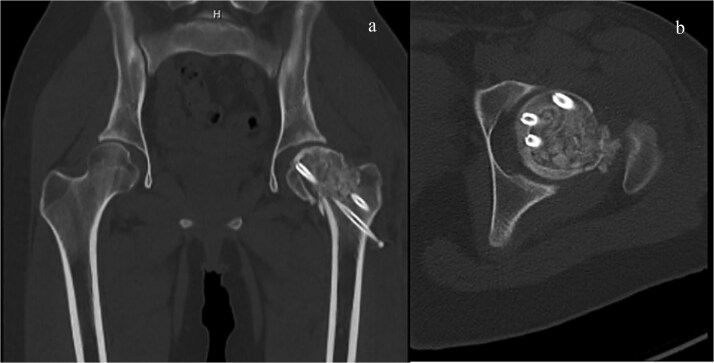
CT imaging after second surgery. The bone defect was filled thoroughly with autologous iliac bone and frozen allogeneic bone. (a) Sagittal view and (b) coronal view.
